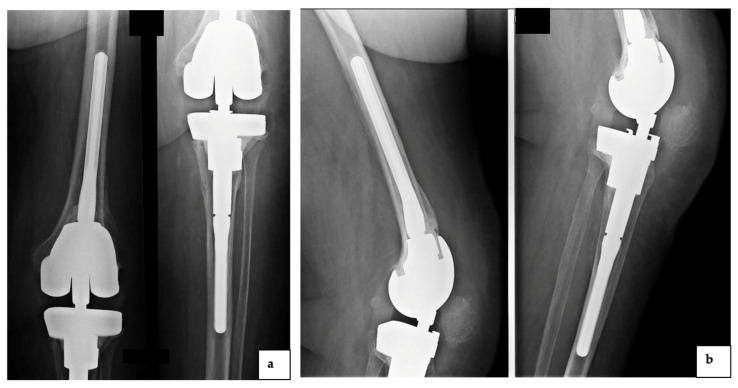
The plain radiograph showed periprosthetic infection of the rotating hinge knee implant due to *Abiotrophia defectiva* ((a): anteroposterior view and (b): lateral view).
